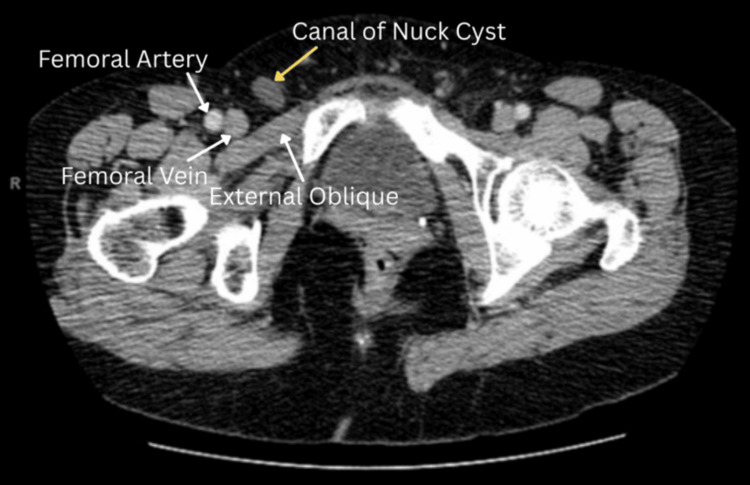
CT of the Abdomen and Pelvis With an Intravenous Contrast of a Canal of Nuck Cyst A CT of the abdomen and pelvis with contrast shows a canal of Nuck cyst (yellow arrow), identified as a non-enhancing, well-circumscribed ovoid mass CT: computed tomography
